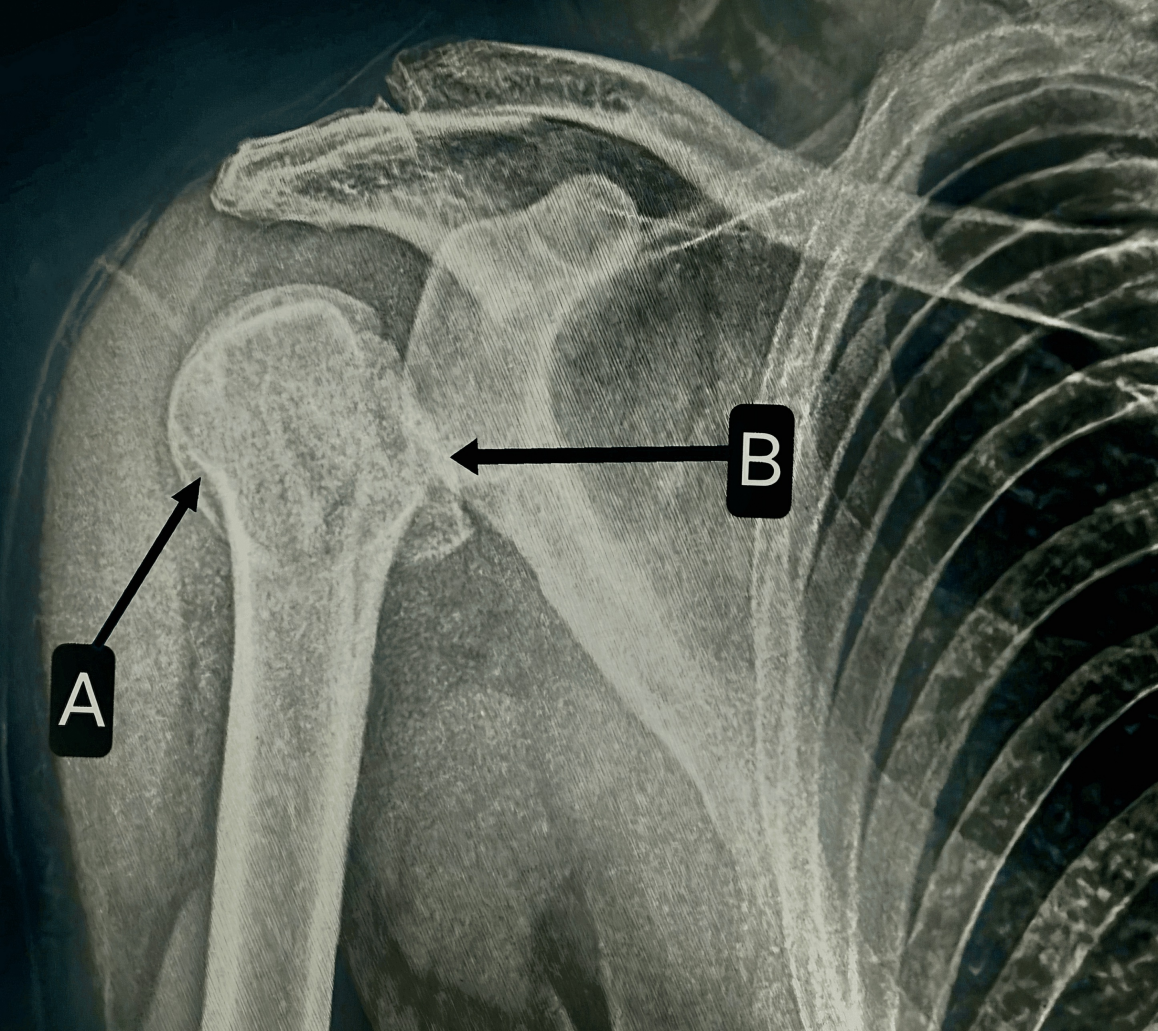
X-ray of the right shoulder showing GT fracture (A) and LT fracture (B) with a posterior dislocation of the shoulder. GT, greater tuberosity; LT, lesser tuberosity
